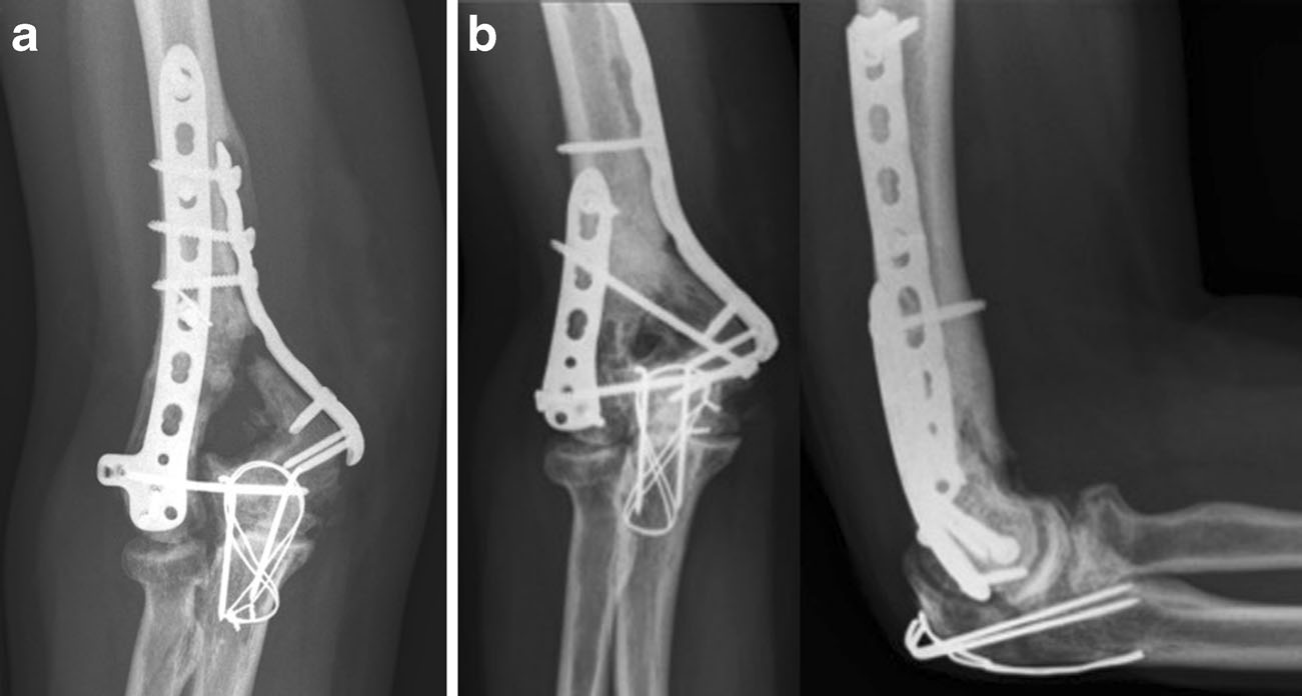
Anatomic locking plates (3.5 mm proximal and 2.7 mm distal) provide an increased number of fixation options. This patient had a nonunion of her distal humerus fracture that showed positive cultures for *Enterobacter cloacae* (a). Revision internal fixation with new plates bone graft and antibiotics resulted in healing as demonstrated on AP and lateral radiographs (*from left to right*); (b).
